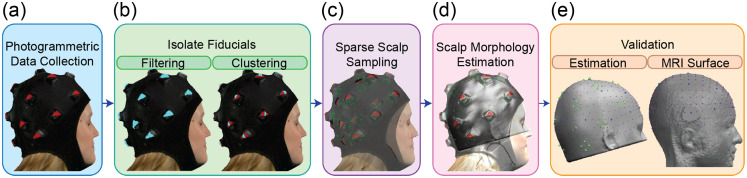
Combination of hardware and algorithm provides robust scalp shape estimation through hair. (a) Participant 3D scan after loading into MATLAB. (b) Nodes that passed color filtering depicted in cyan (left). K-means clustering isolates individual fiducials of filtered points into 17 clusters (centroids of fiducials shown in cyan, right). (c) Sparse scalp sampling (green) overlaid with 3D scan. (d) Scalp morphology estimation using the MNI152 atlas overlaid with the 3D scan and sparse scalp sampling. (e) The international 10 to 20 (blue) and modified 10 to 5 (purple) on the scalp surface estimation with sparse scalp sampling (green, left) and on participant-specific MRI (right).
